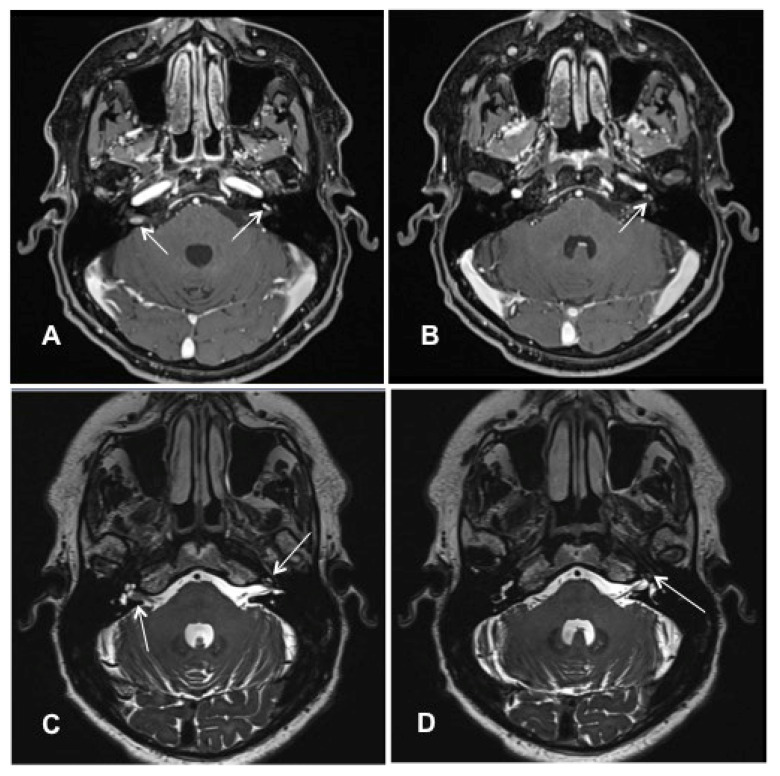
Case #4: MR images visualizing bilateral ILS (right side: intrameatal location, left side: intracochlear location). (A,B): T1 weighted VIBE 3D with fat saturation prepulse after the intravenous administration of gadolinium. (A) White arrows: intrameatal VS on the right side and ILS on the left side. (B) The white arrow points to the intracochlear schwannoma on the left side (middle turn); (C,D): T2 weighted SPACE sequences. (C) White arrows point to the intrameatal VS on the right and the ILS on the left. (D) The white arrow points at the ILS on the left side (middle turn).
